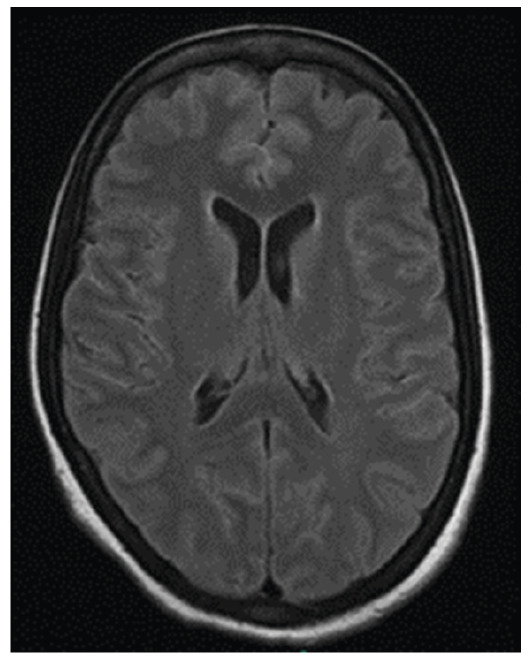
Head MRI revealing T2/FLAIR hyperintensities at the surface of the brain gyri and ependyma.
